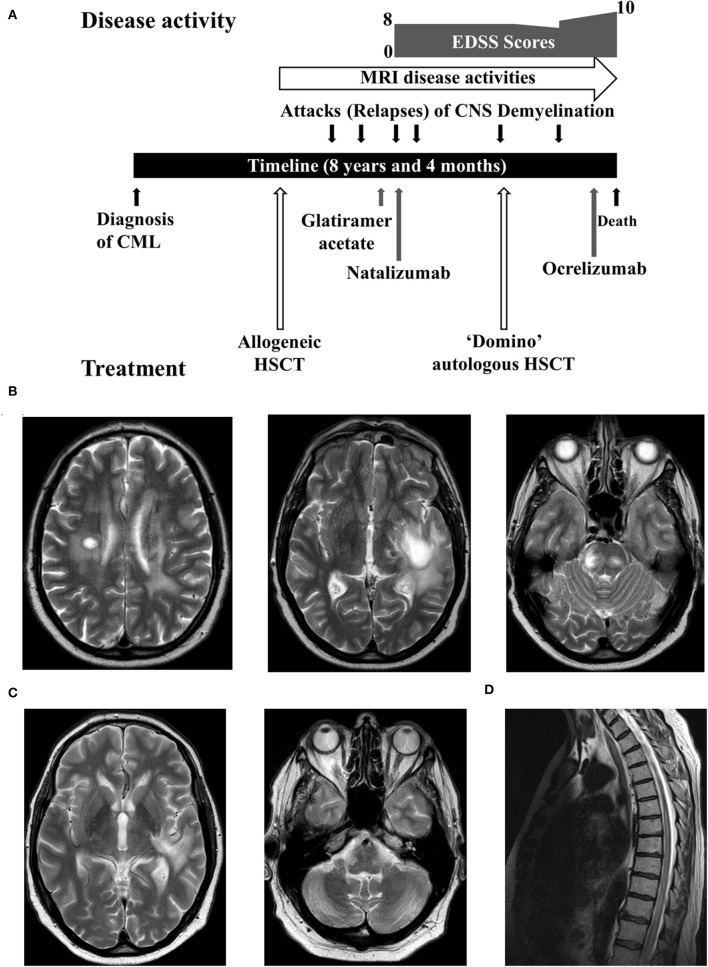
(A) A summary of the disease course. (B) Axial sections of the brain MRI showed tumefactive lesions. (C) Improvement of the edema around tumefactive lesions. (D) The spine MRI demonstrated a lesion extending from the thoracic cord to conus.
